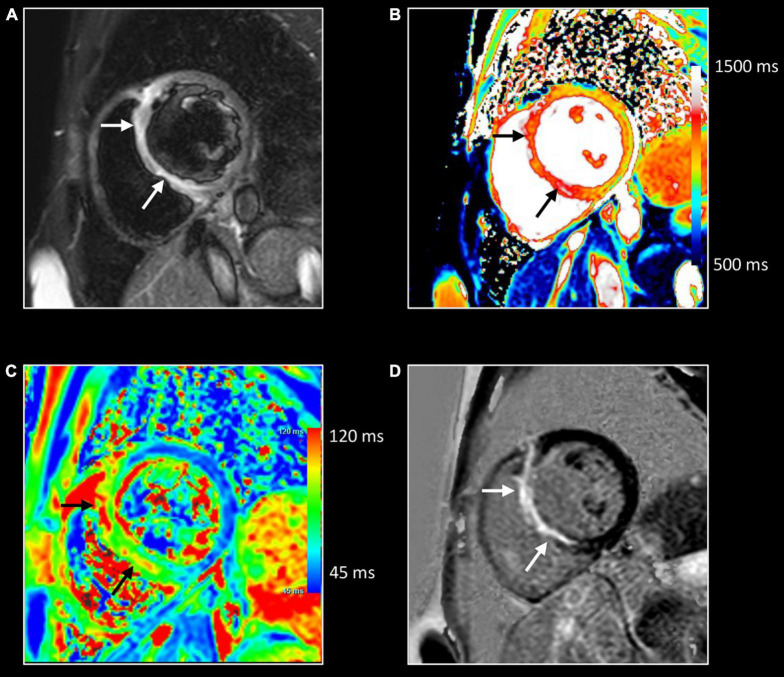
Giant cell myocarditis rapidly evolving to heart failure. Forty-six-year-old woman presenting with monomorphic ventricular tachycardia arising from the basal septum. Transthoracic echocardiography shows mildly decreased LV function (EF 49%) with dyskinetic basal septum. CMR shows mildly dilated and dysfunctional left ventricle (LVEDVi 107 ml/m2 – LVEF 45%), and thinned, dyskinetic basal septum. T2w-imaging (A) shows myocardial edema in the basal part with strong increase of native T1 (i.e., 1,230 ms) and T2 (i.e., 99 ms) relaxation times in this area [arrows (B,C), respectively]. Strong uptake of contrast showing a non-ischemic enhancement pattern but in a large extent completely transmural [arrows, (D)] and increased ECV (i.e 65%) (not shown). Findings suspected of active inflammation in the basal septum with local adverse remodeling. A left-sided myocardial biopsy showed giant cell myocarditis. Follow-up CMR, one month later, showed important deterioration of LV function (LV EF 26%). Because of the unfavorable outcome, the patient received a cardiac transplant. Two years later, she is doing well.
